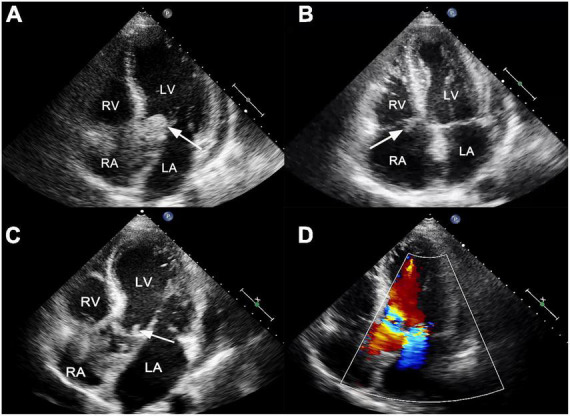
(A) Transthoracic echocardiogram showed a large, mobile vegetation on the ventricular side of the prosthetic valve (white arrow). (B) A vegetation was also visible on the tricuspid valve (white arrow). (C) Transthoracic echocardiogram reexamination showed residual vegetations on prosthetic aortic valves with reduced size (white arrow). (D) The new mitral valve moderate regurgitation. LA, left atrium; LV, left ventricle; RA, right atrium; RV, right ventricle.
